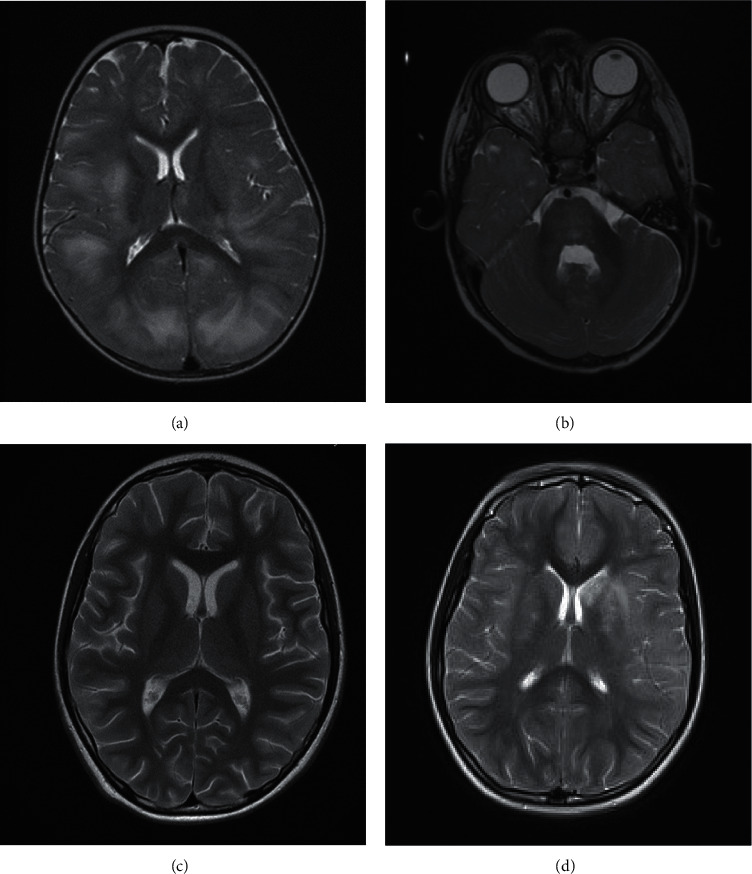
(a, b) MRI brain of Case 1 showing bilateral T2 hyperintensities of the subcortical white matter of all the brain and splenium of the corpus callosum with associated diffusion restriction and signal change in thalami and pons. (c) Case 2: normal MRI brain on acute presentation. (d) Repeat MRI brain 50 days later showing bilateral T2 hyperintensity of the basal ganglia and parasagittal frontal lobes, anterior limb of the left internal capsule, insula, and subcortical white matter regions.
